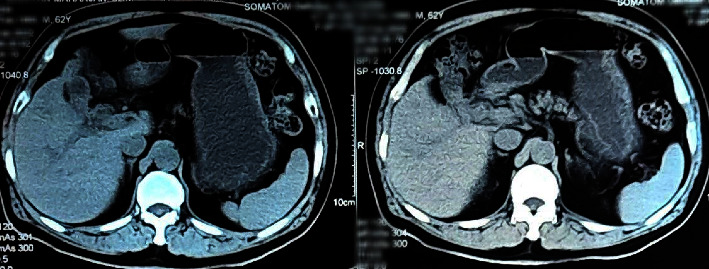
Contrast-enhanced computed tomography (CECT) abdomen pelvis showing partially distended gallbladder with thickening at the region of the body with luminal narrowing.
